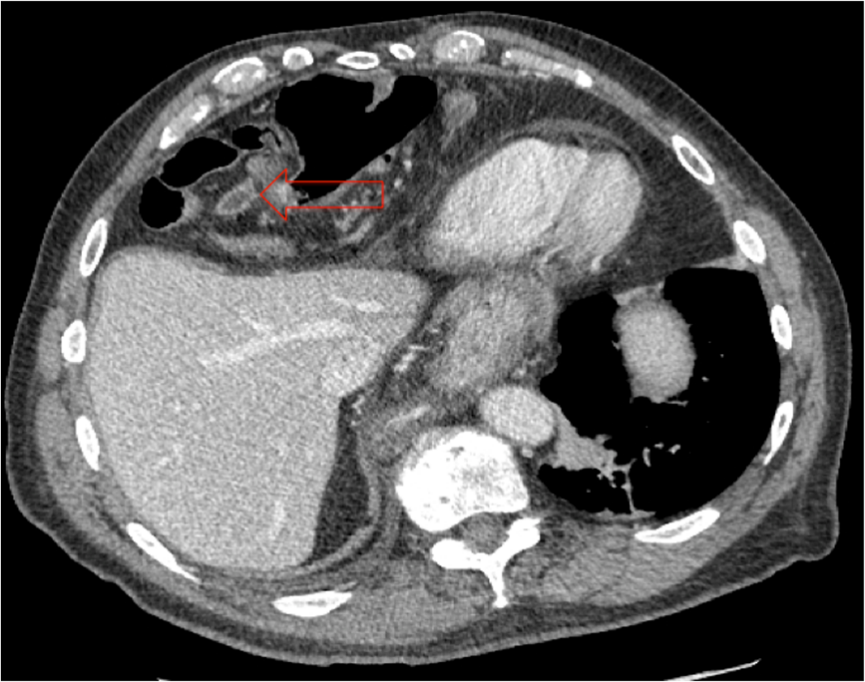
Computed tomography scan obtained at our institution 8/2013, with arrow showing appendiceal inflammation within Morgagni defect. Computed tomography scan obtained at our institution 8/2013, with arrow showing appendiceal inflammation within Morgagni defect.
